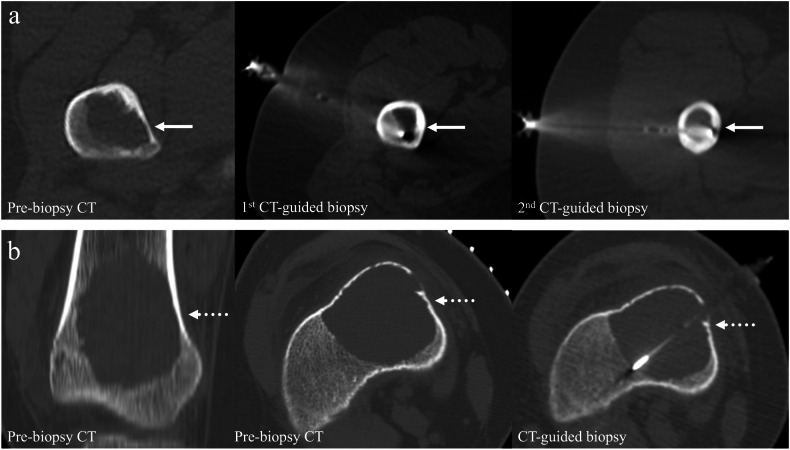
Examples of benign bone lesions with non-diagnostic CT biopsy. a Initial CT-guided biopsy of the proximal femoral lytic lesion (solid arrows) was non-diagnostic. Fibrous dysplasia was confirmed in the second CT-guided biopsy. b CT-guided biopsy of a distal femoral lytic lesion involving the epiphysis (dotted arrows) was non-diagnostic. Curettage was subsequently performed, and a giant cell tumor was confirmed pathologically
